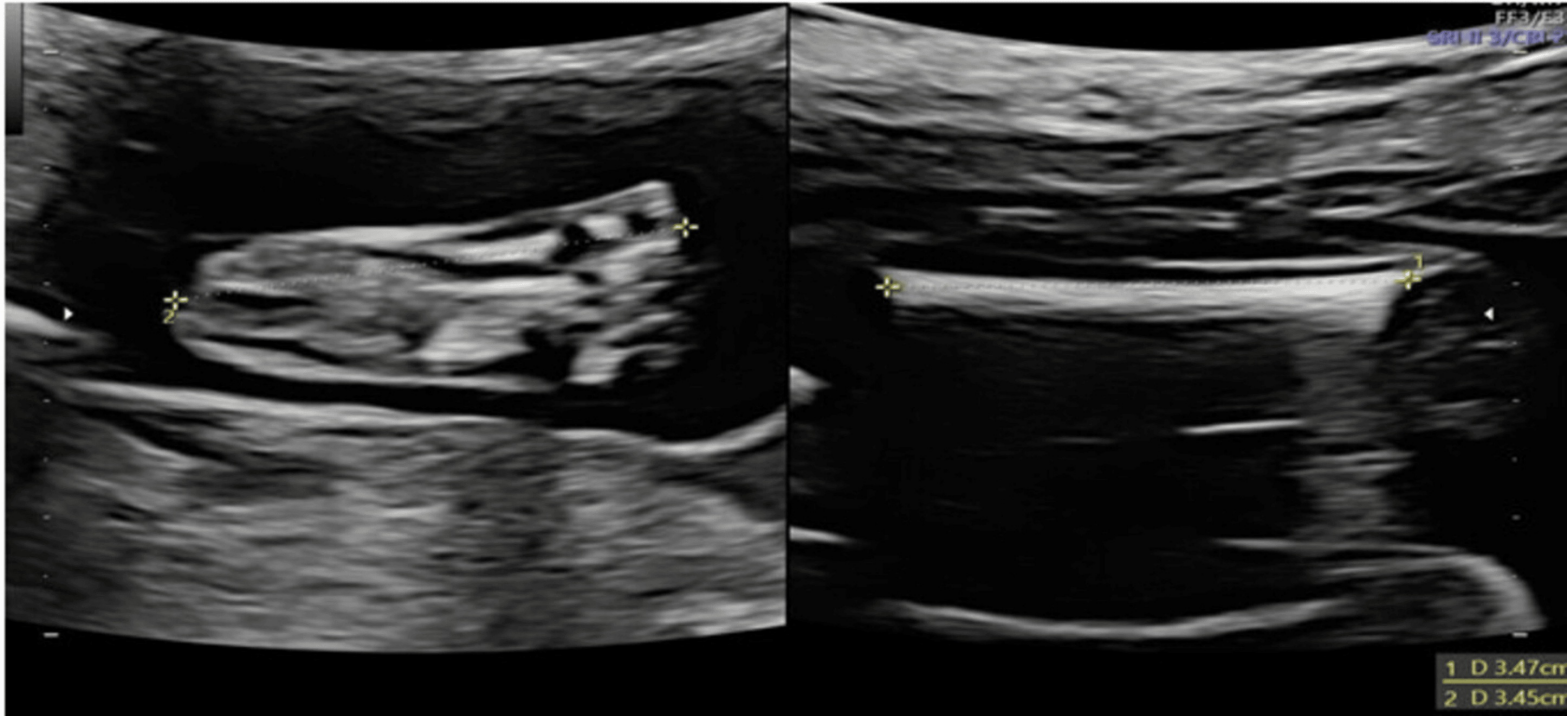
Ultrasonography (USG) image showing the foot (plantar view) and femur.
